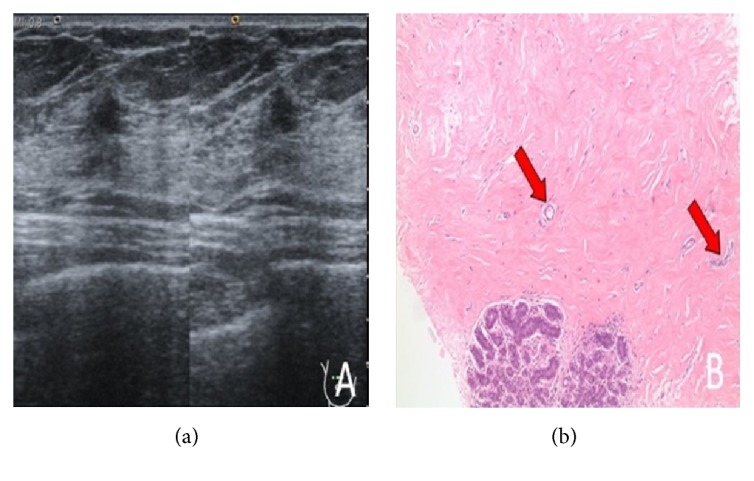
41-year-old woman presenting for screening. Mammography (not shown) demonstrated heterogeneously dense breast with no suspicious abnormality. (a) Screening ultrasound shows a hypoechoic mass with irregular shape and margin. The patient was lost to follow-up after the biopsy. (b) Dense fibrous stroma with small capillaries (arrows) and adjacent appearing lobular units.
